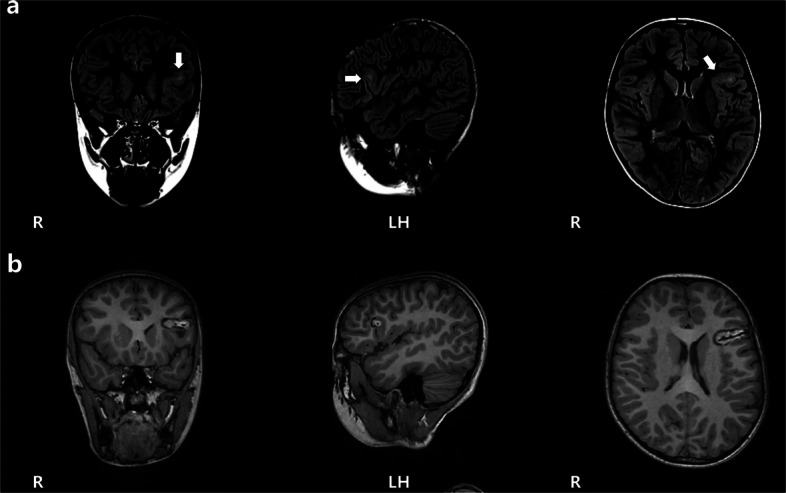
a shows abnormal signals in the opercular part of the left IFG on the coronal, sagittal and axial view of MRI. b shows the majority of the epileptogenic focus had been destroyed
